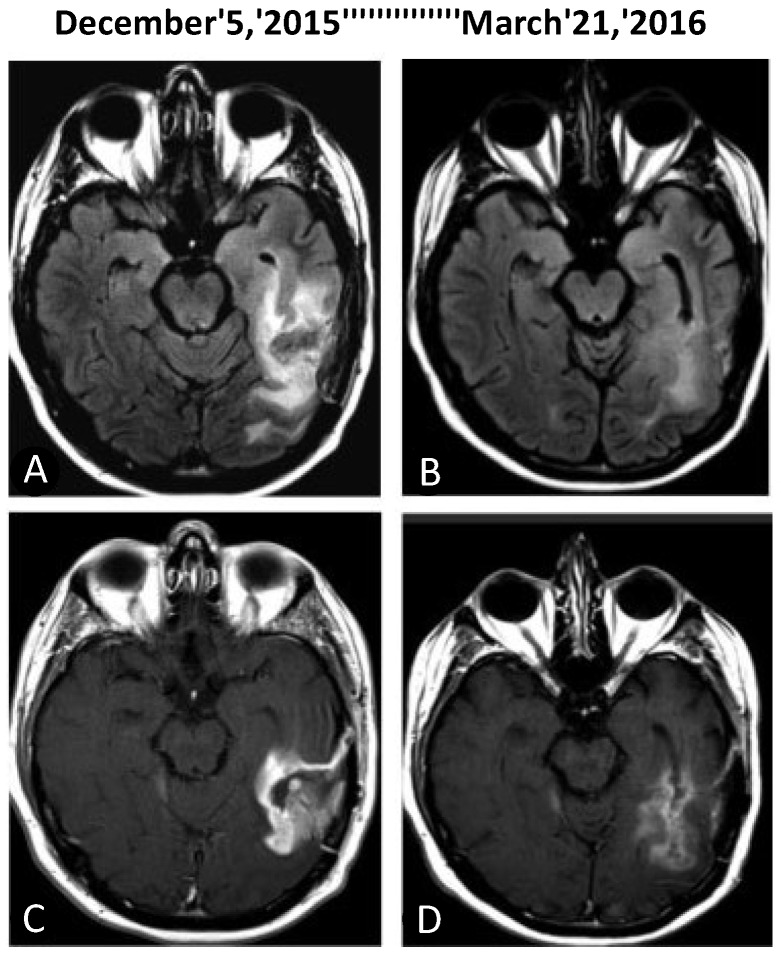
Effect of KD concomitant with inhalational POH in a patient with recurrent GB. Representative brain MRI scans are shown from before the onset of treatment (left panels) and after 3 months of treatment (right panels). (A,B) MRI axial fluid-attenuated inversion recovery (FLAIR); (C,D) T1-weighted image (T1W) with contrast. Note marked reduction of peritumoral edema and tumor size after treatment.
